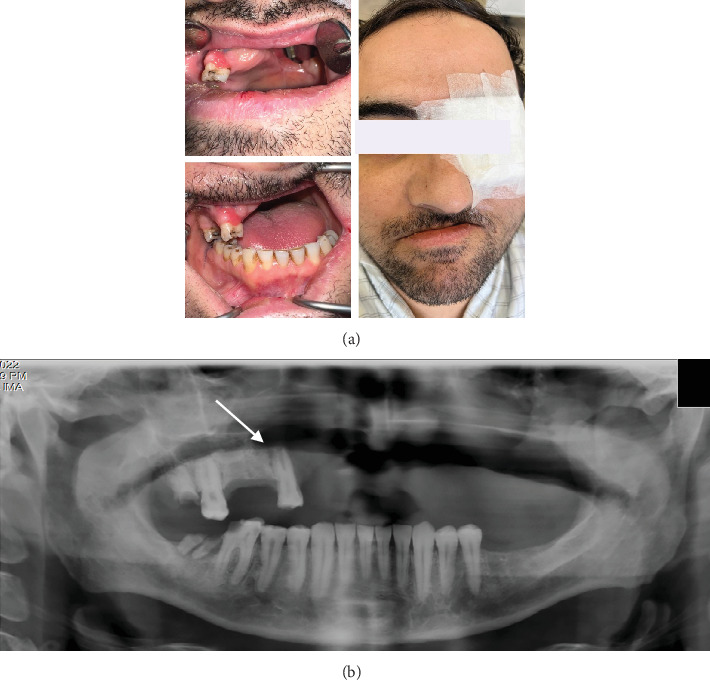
(a) Intraoral and extraoral photographs of the patient who had a history of hemimaxillectomy. The left orbit was exenterated due to the spread of the fungal infection. (b) Panoramic radiograph with the arrow pointing to bone destruction around the right maxillary premolar, suggesting recurrence of the disease.
